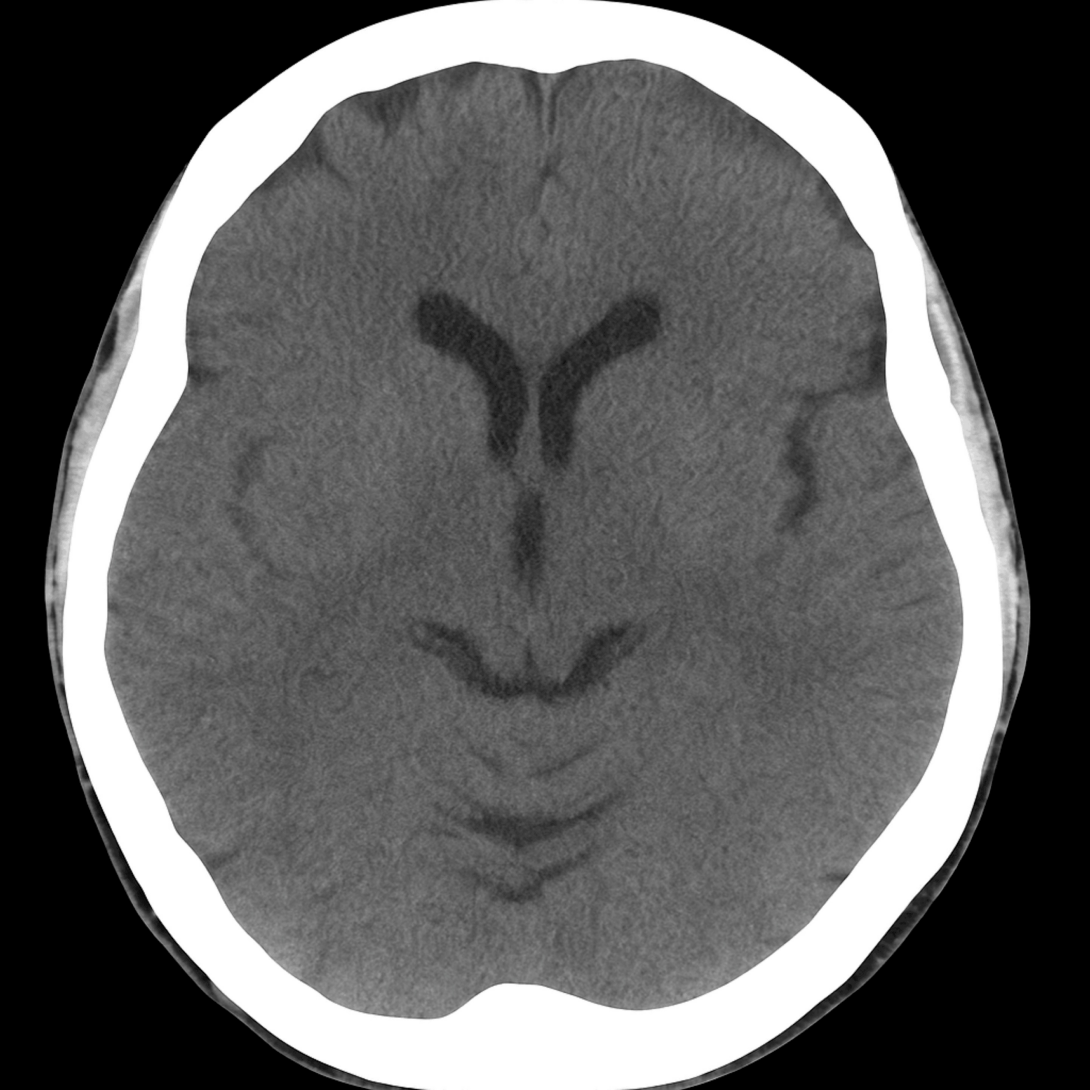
Non-contrast CT head demonstrating no evidence of acute intracranial pathology: no haemorrhage, infarction, mass effect, or ischaemic changes are seen. This imaging was performed to exclude neurovascular complications such as stroke or intracranial vasculitis, which can occasionally accompany LVV.
